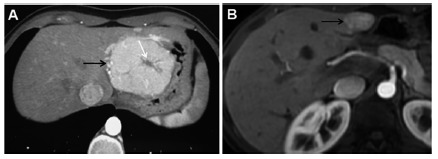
A) Arterial phase of a CT exam in a patient with typical FNH (black arrow) where a hypodense central scar can be observed in the center of the lesion (white arrow); B) arterial phases of MRIs in young patients with atypical arterialized lesions (white arrows) in steatotic liver where homogeneous emphasis of the lesions can be observed; control examinations at 24 months showed stability of the lesions, which were therefore considered "most likely benign"
